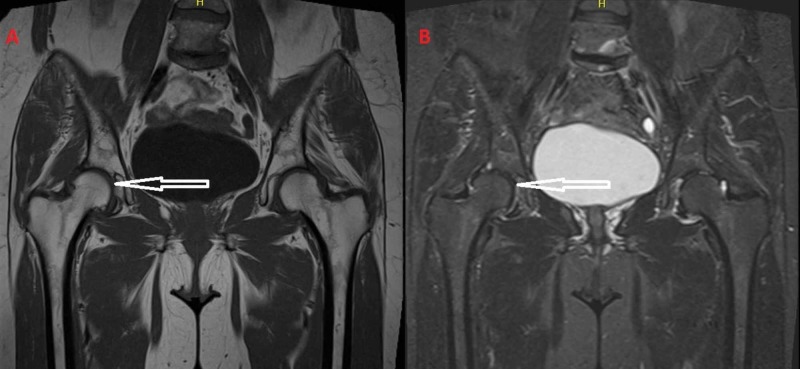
Regression of the bone marrow edema is evident at follow-up MRI (A: T1-weighted images and B: T2-weighted images).
